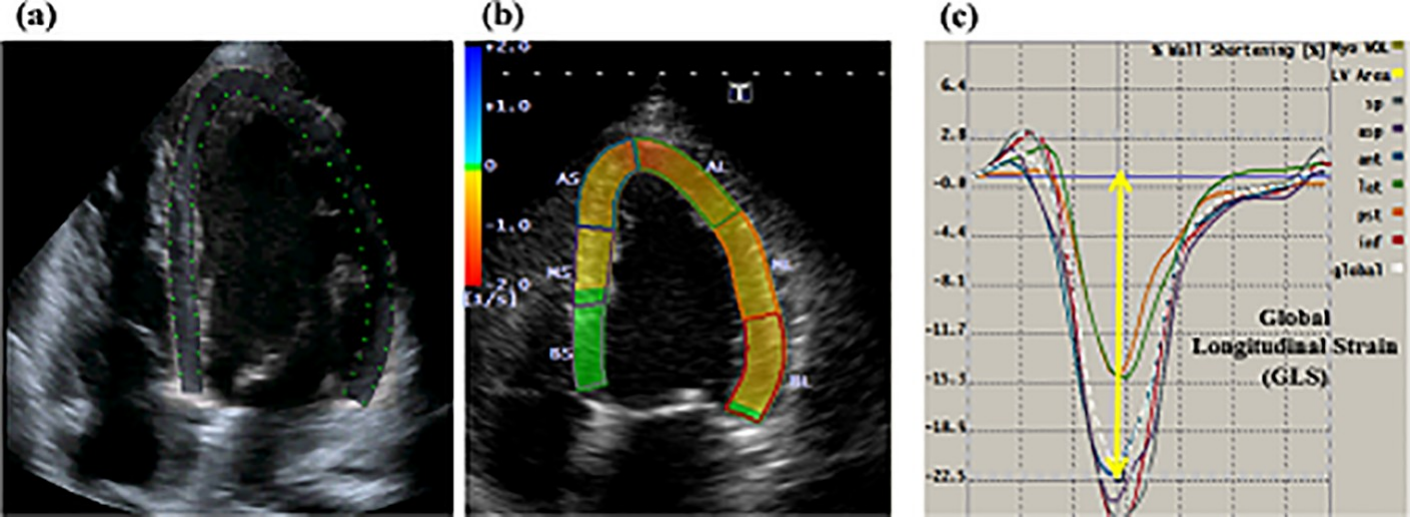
Example of the measurement of left ventricular (LV) global longitudinal strain (GLS) with a two-dimensional speckle-tracking echocardiography (2DSTE) in a patient with chronic total occlusion who underwent successful percutaneous coronary intervention (PCI). Figure (a) and (b) showed apical four-chamber view. White broken lines indicated the LV global strain curves for GLS. Yellow arrow indicated the mean LV global peak longitudinal strain; GLS: −22.5% (c).
